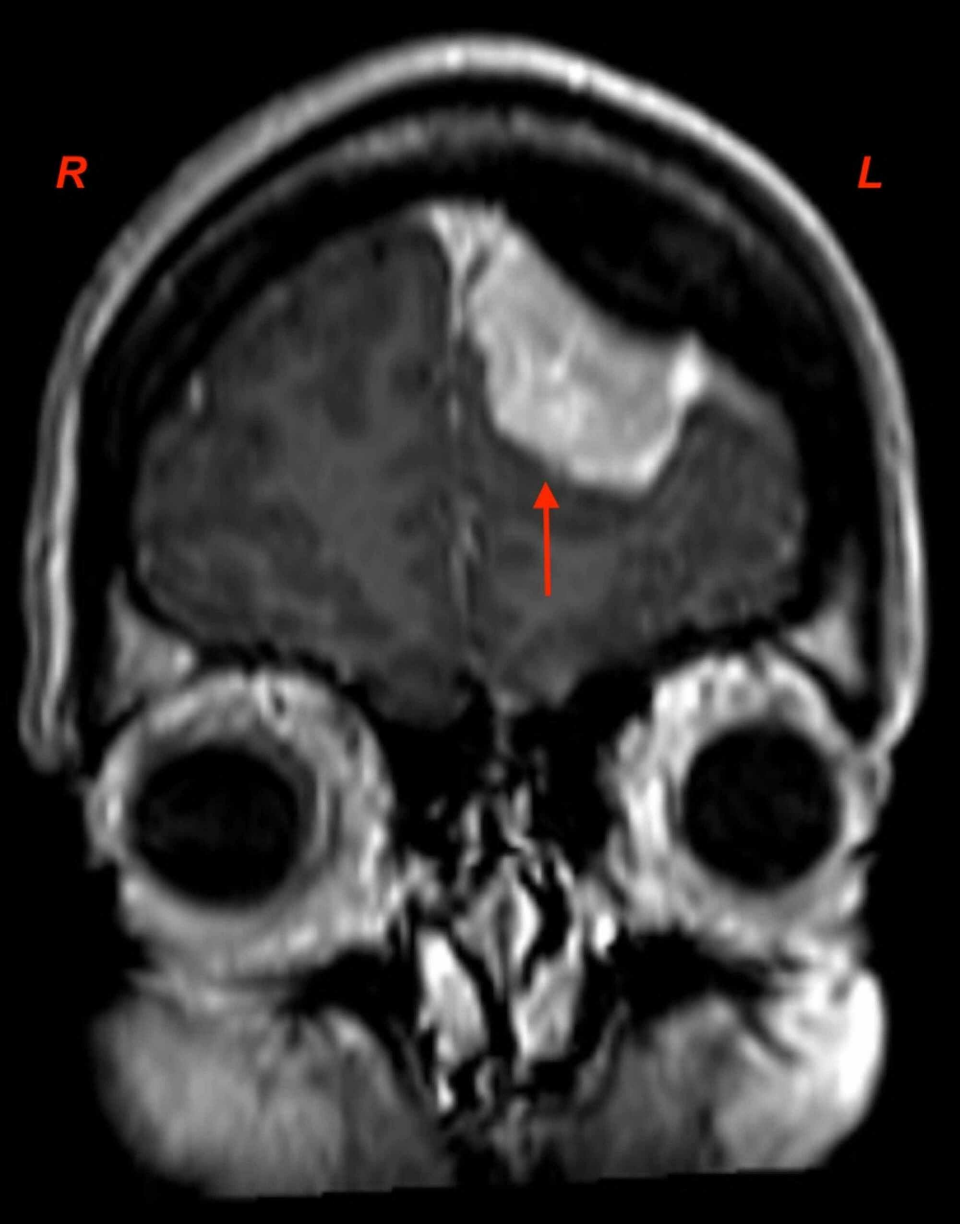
Coronal T1-weighted MRI-contrast scan demonstrating the left-sided, frontal meningioma. Parafalcine expansion is visible at depth.
